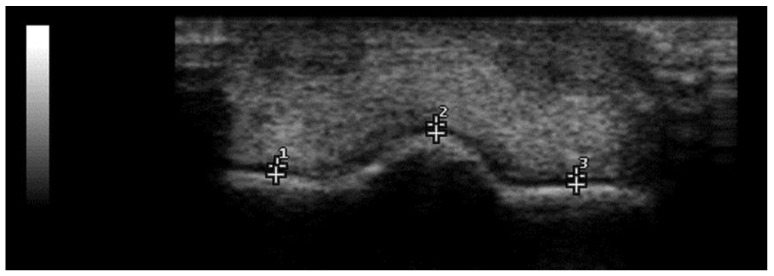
Transversal ultrasound view of thoroughbred MCP joint. (1) Lateral condyle, (2) sagittal ridge and (3) medial condyle. Lateral is to the left. B-mode, linear probe, 7.5 MHz.
